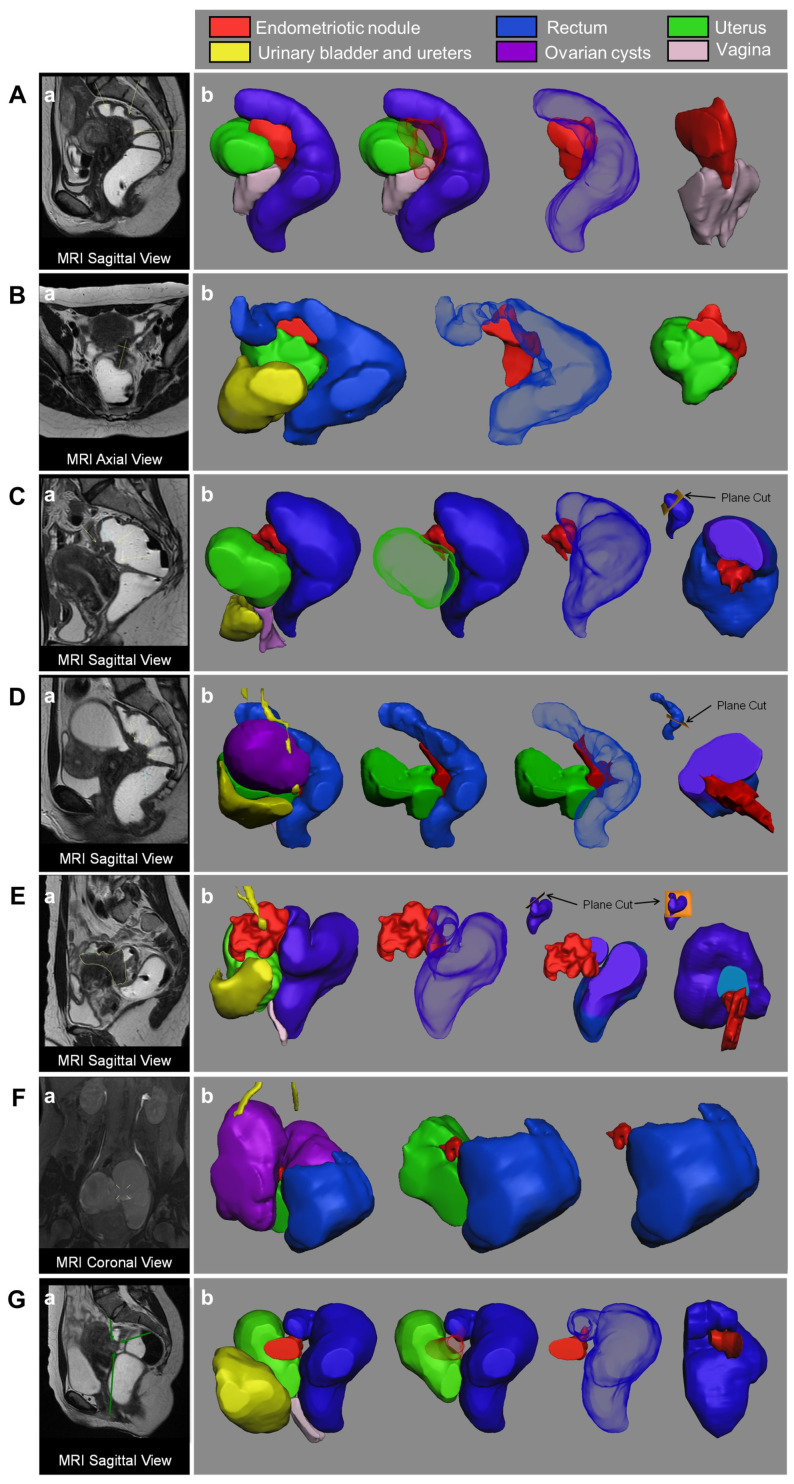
Two-dimensional MRI images (a) and 3D models views (b) of the 7 included women. The 3D anatomic reconstruction of the recto-sigmoid endometriosis (RSE) nodule with the surrounding pelvic organs is presented, demonstrating their spatial relationship, as well as the isolated nodule to show the nodule’s extension (A–G). The cutting plane is shown in cases C, D and E.
